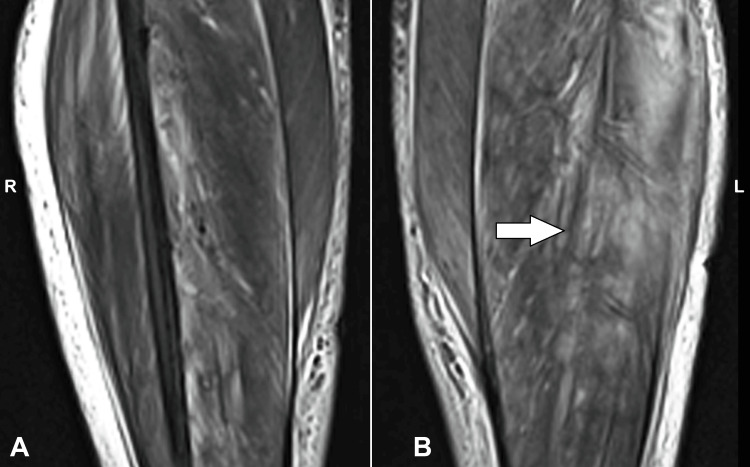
MRI axial section showing affected areas of the (A) right and (B) left muscles (white arrow) MRI: Magnetic resonance imaging
